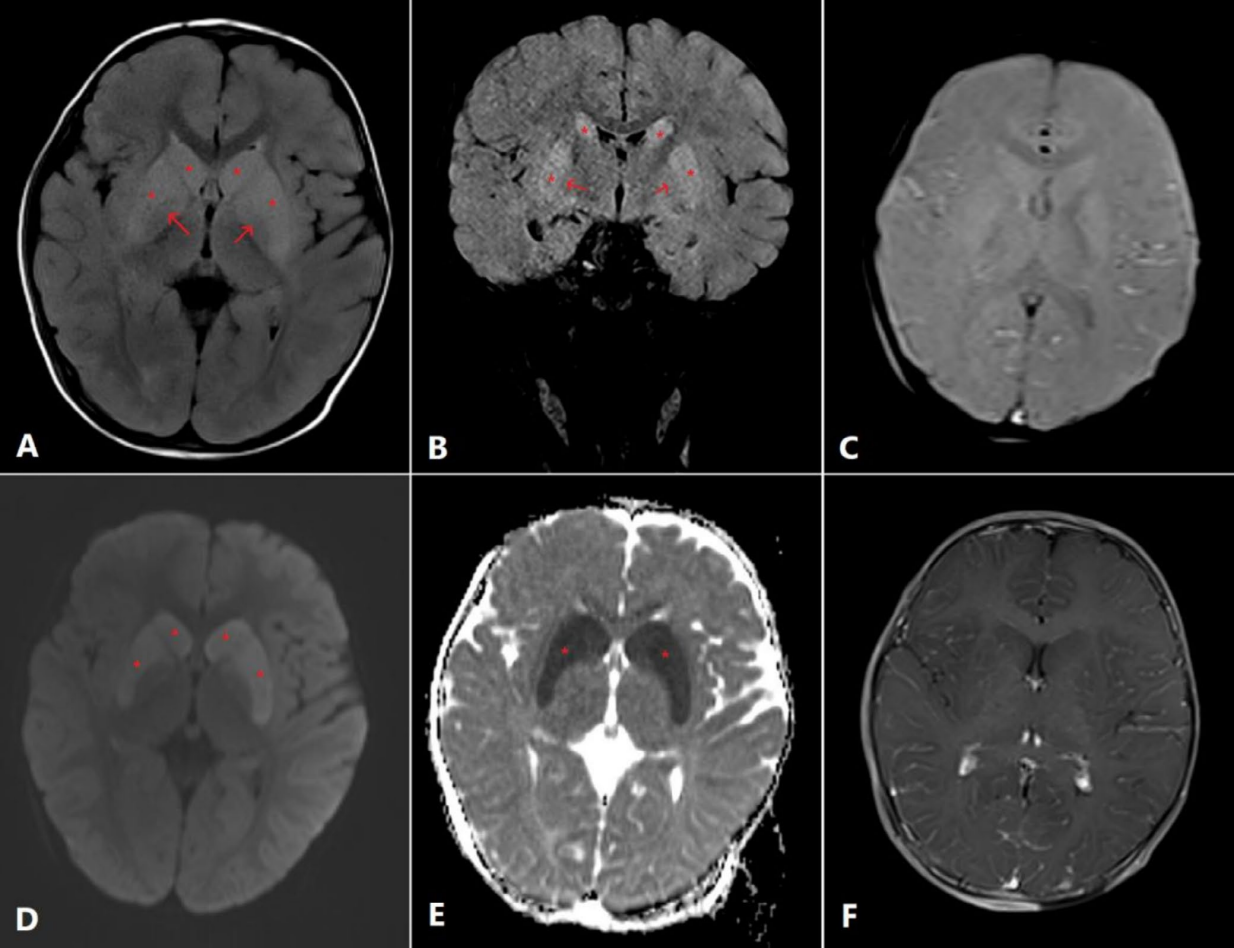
(A, C–F) Selected axial and (B) coronal cuts of the patient's MRI [A: Axial Fluid attenuated inversion recovery (FLAIR) image, B: Coronal FLAIR image, C: Axial Susceptibility weighted imaging (SWI), D: Diffusion‐weighted image (DWI), E: Apparent diffusion coefficient (ADC), F: Axial T1 fat sat/FS with contrast]. There is bilateral symmetrical increased T2/FLAIR signal and edema involving the lentiform and caudate nuclei (asterisks in A and B) with relative sparing of the globus pallidus (solid arrows in A and B) associated with diffusion restriction (high DWI signal—asterisks in D, with corresponding low ADC value—asterisks in E). No associated postcontrast enhancement (F) or blooming artifact on the SWI (C).
